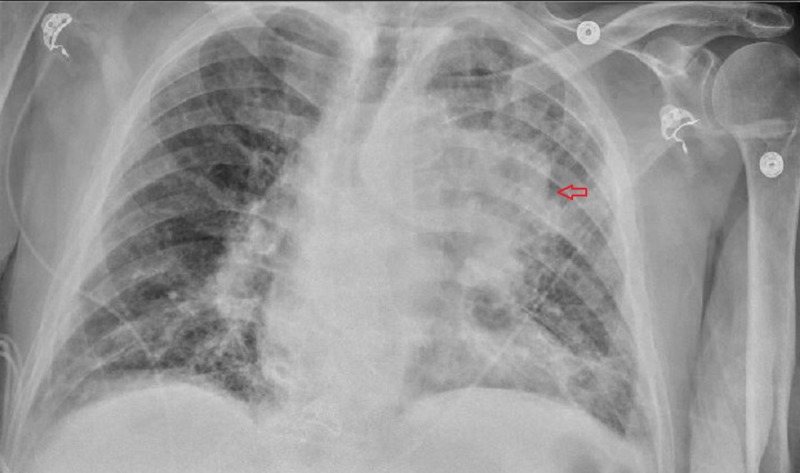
Chest X-ray showing left hilar and suprahilar opacity (arrow) with adjacent lung atelectasis
